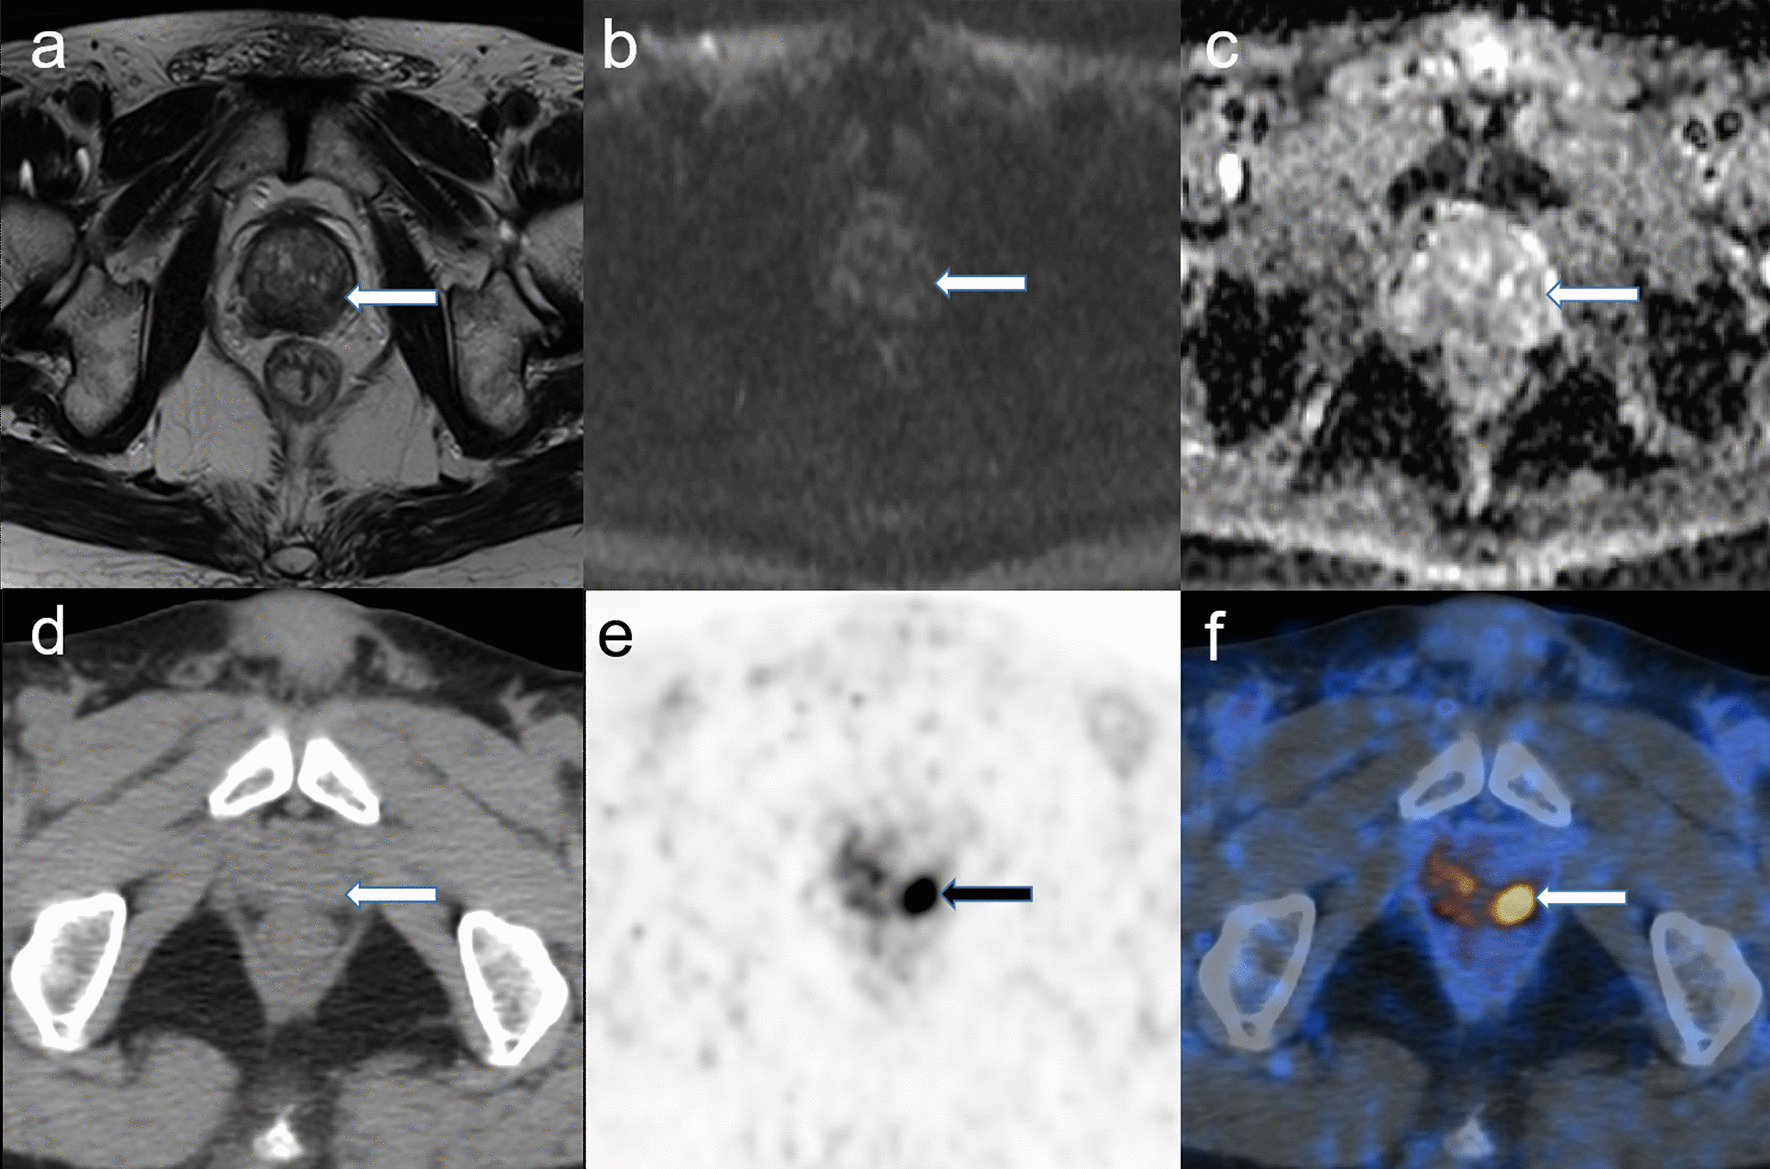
Low- and intermediate-risk PCa with PSA > 9.4 ng/ml and age > 62.5 years, who are mpMRI (−) and ^68^Ga-PSMA PET/CT (+). A 73-year-old patient whose serum PSA level was 17.9 ng/ml and whose GS was 3 + 4. There is no lesion in the prostate gland in the pelvic MRI scan (a T2w; b b 1500 DWI), while in the ^68^Ga-PSMA PET/CT, there is a strong tracer uptake highly likely to be diagnosed (arrow in e and f). a–c MRI; d–f
